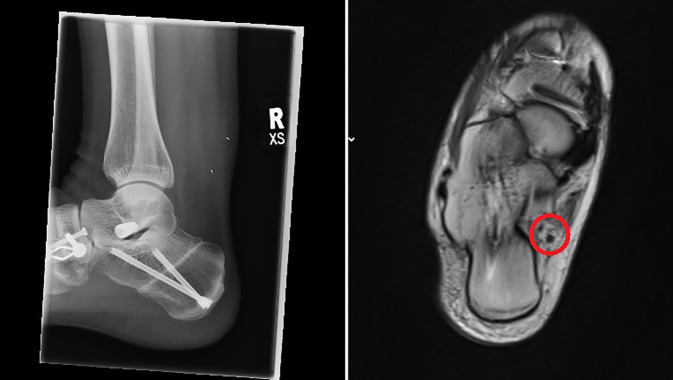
X‑ray lateral (*left*) and MRI axial images (*right*) after flatfoot reconstruction. Even though the calcaneal osteotomy does not seem to be excessively too distal, the neurovascular bundle medially is at risk (*red circle*)
